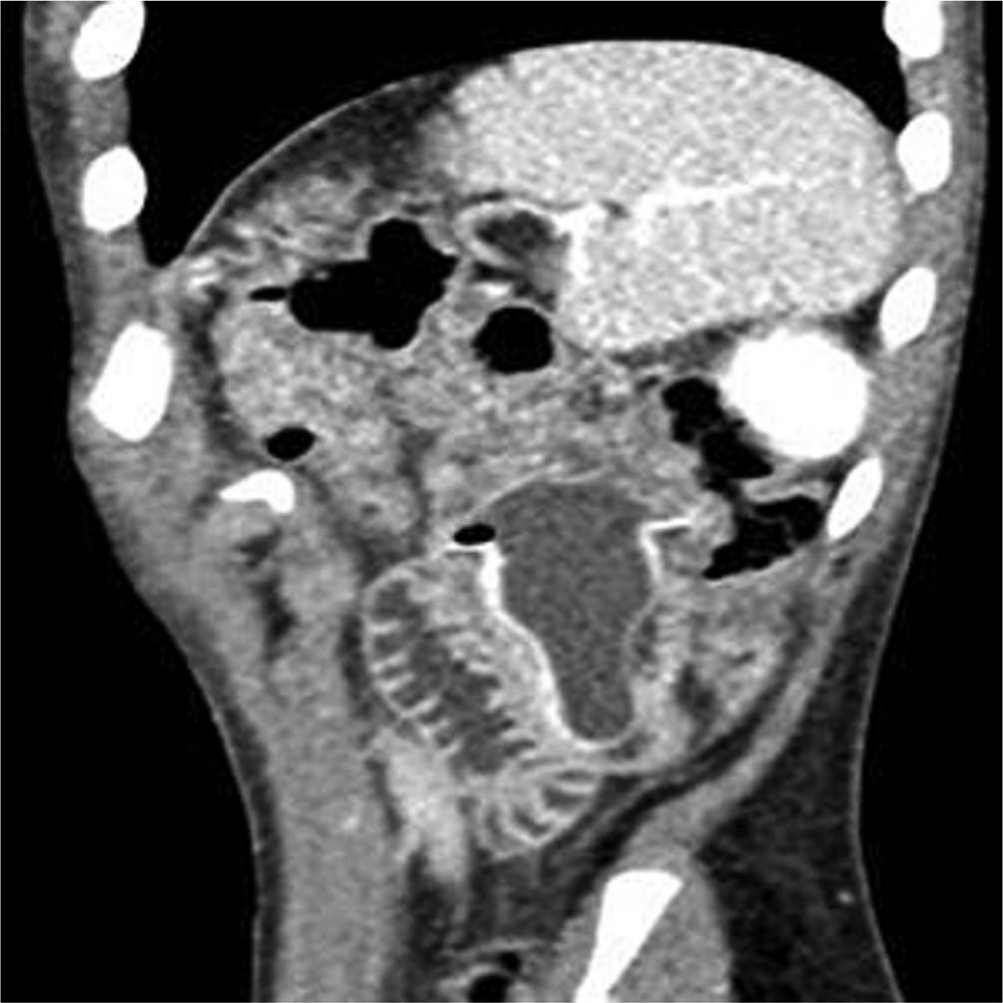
CT sagittal view showing fluid-filled nipple of feeding bottle lodged in jejunum.
